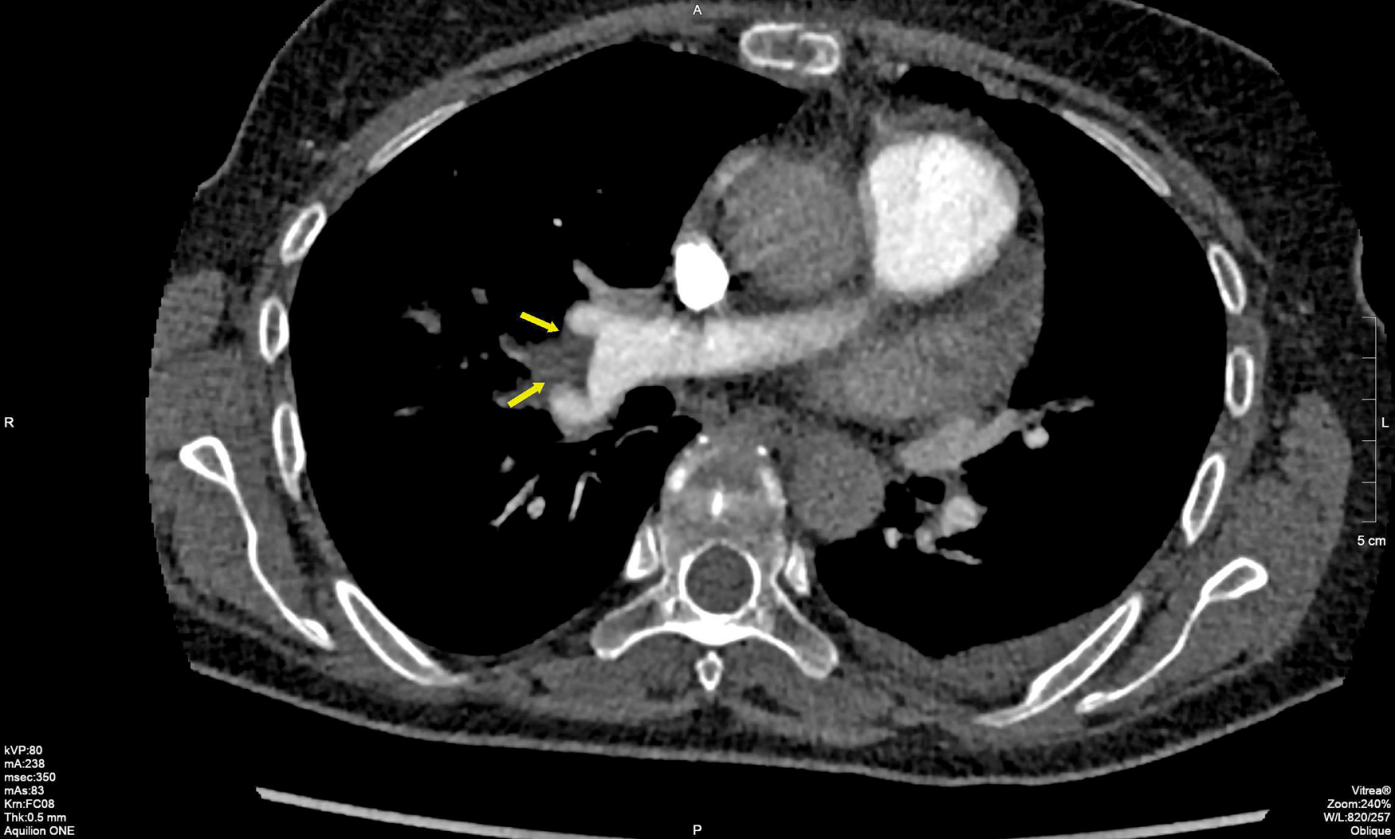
MSCT of the thorax showing small residual thrombotic masses. MSCT, multi-slice CT.
